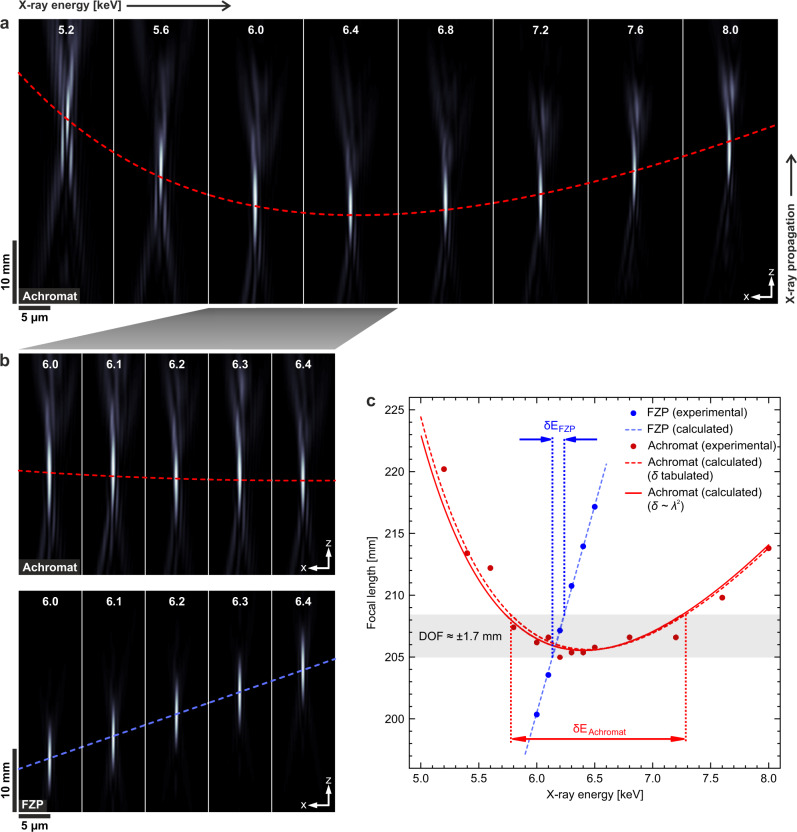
Evolution of the X-ray beam profile with the energy measured with ptychography. a Caustics at energies from 5.2 keV to 8.0 keV obtained with the achromat. The red dashed line indicates the location of the focal plane at the different energies. b Comparison of the caustics obtained with the achromat and the FZP. While the position of the focal plane remains almost constant with the energy for the achromat (red dashed line), it changes rapidly for the FZP (blue dashed line). c Calculated curves (solid and dashed lines) and experimental data (dots) for the focal length versus energy for the FZP (blue) and the achromat (red; solid: based on Eq. (6), dashed: based on tabulated refractive index values for the calculation of *f*_RL_ at each energy).
